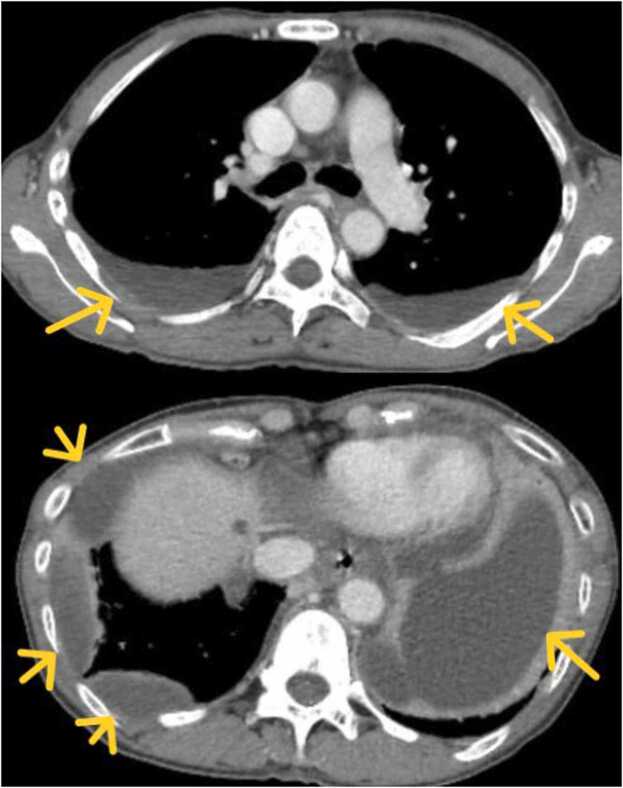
Computed tomography scans(CT) on admission. That revealed bilateral posterior and basal pleural effusions (yellow arrows).
